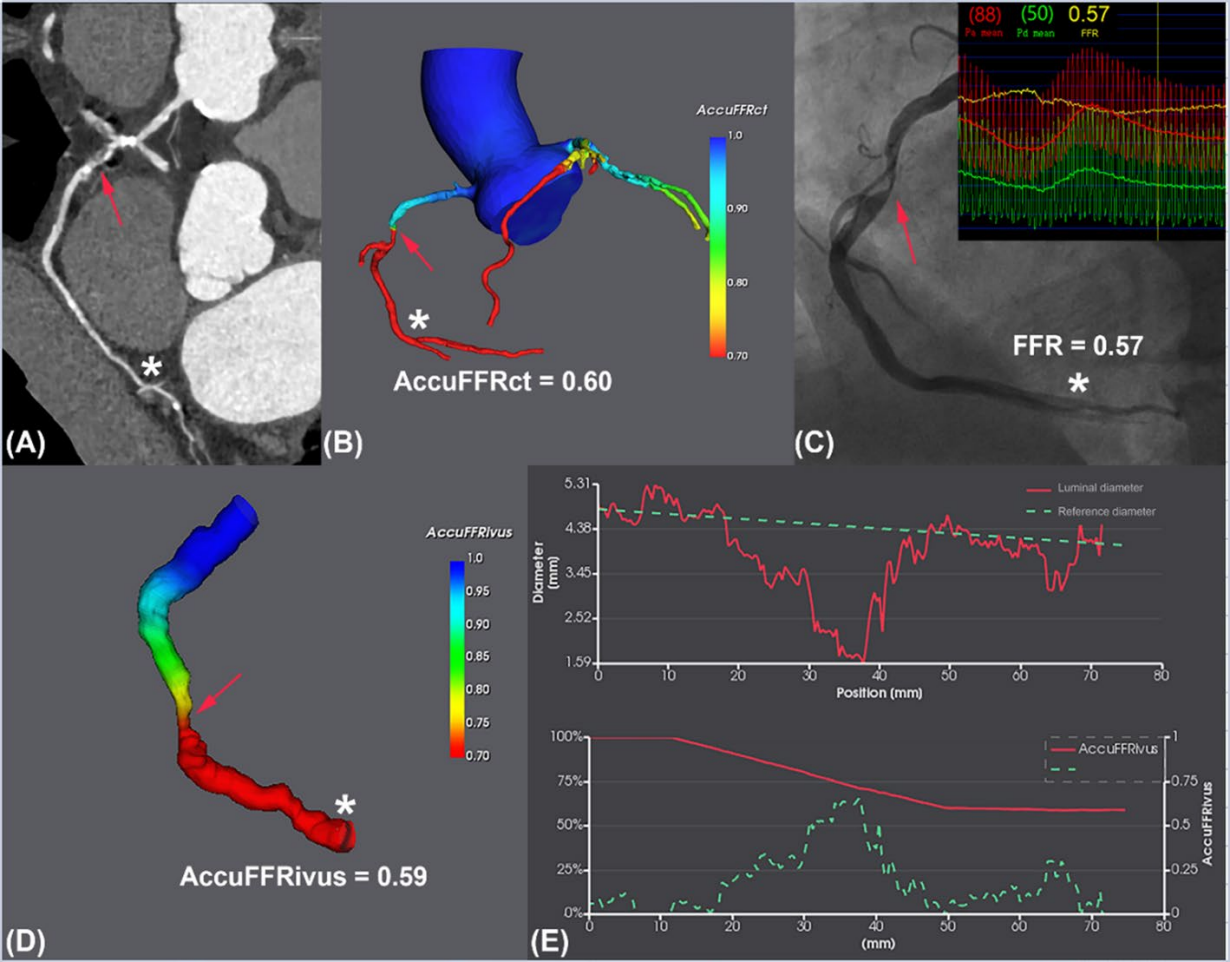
A representative example of AccuFFRct, AccuFFRivus, and FFR measurements. A Computed tomography angiography (CTA)-derived percentage diameter stenosis (%DS) = 60%. B Fractional flow reserve derived from computed tomography (AccuFFRct) = 0.60. C Wire-based fractional flow reserve (FFR) = 0.57. D Fractional flow reserve derived from intravascular ultrasound (AccuFFRivus) = 0.59. E Lumina diameter and AccuFFRivus pull back. Red arrows indicate the stenosis lesion. Asterisks indicate the location of measurement
